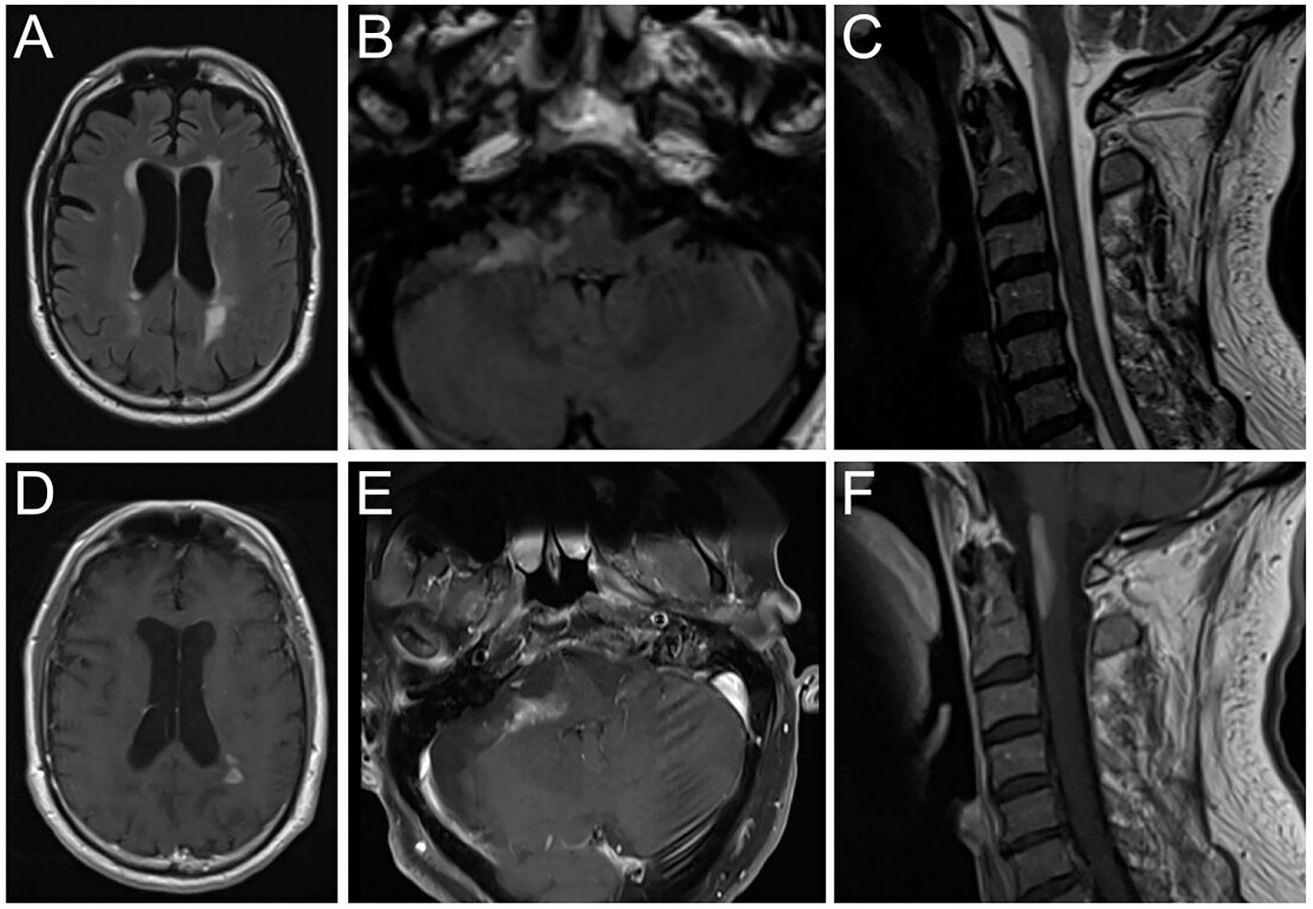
Brain magnetic resonance imaging showing FLAIR hyperintense lesions (A–C) and T1 contrast-enhancement (D–F) in the periventricular white matter (A,D), right cerebellar peduncle (B,E) and medulla oblongata/upper cervical spinal cord (C,F). FLAIR, Fluid attenuated inversion recovery.
